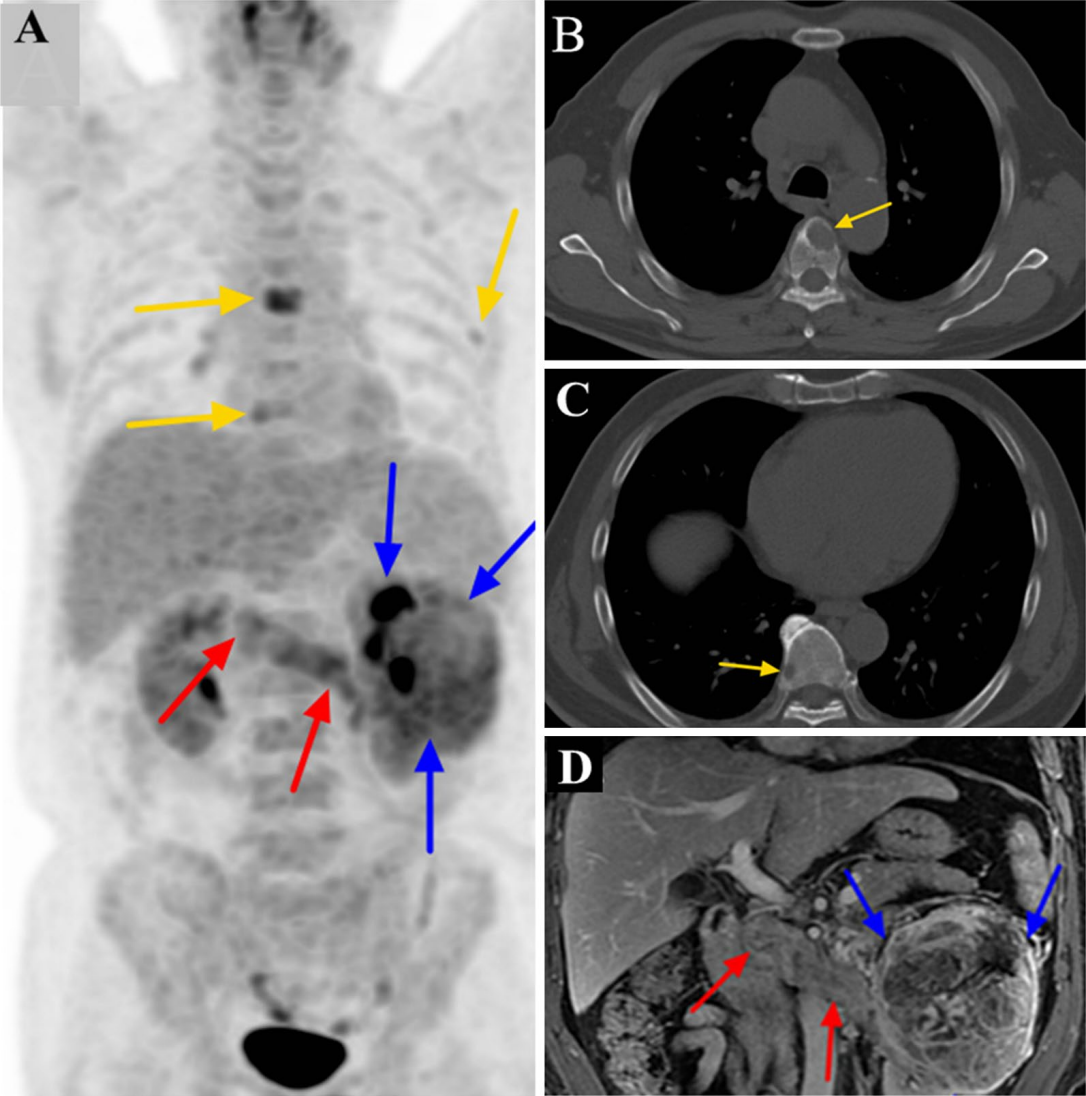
^18^F-FDG PET/CT and contrast-enhanced MRI (CEMRI) scans of a 59-year-old man with clear cell carcinoma of the left kidney associated with a level II venous tumour thrombus (VTT). The VTT invaded the venous wall and was graded as level II by both PET/CT and CEMRI. The site of diffusely increased FDG uptake (SUVmax 4.3) on a PET maximum intensity projection image is the primary tumour (blue arrow) (a). The site of pathologically increased FDG uptake (SUVmax 4.5) on the PET image from the left renal vein to the inferior vena cava is the VTT (red arrow) (a). Some bone metastases in the thoracic vertebrae and a left rib showed increased focal FDG uptake (yellow arrow) (a) with lytic lesions on selected axial CT slices (yellow arrow) (b,c). The VTT did not show homogeneous enhancement and completely occluded the lumen of the inferior vena cava on coronal CEMRI (red arrow) (d). The primary tumour showed heterogeneous enhancement (blue arrow) (d).
